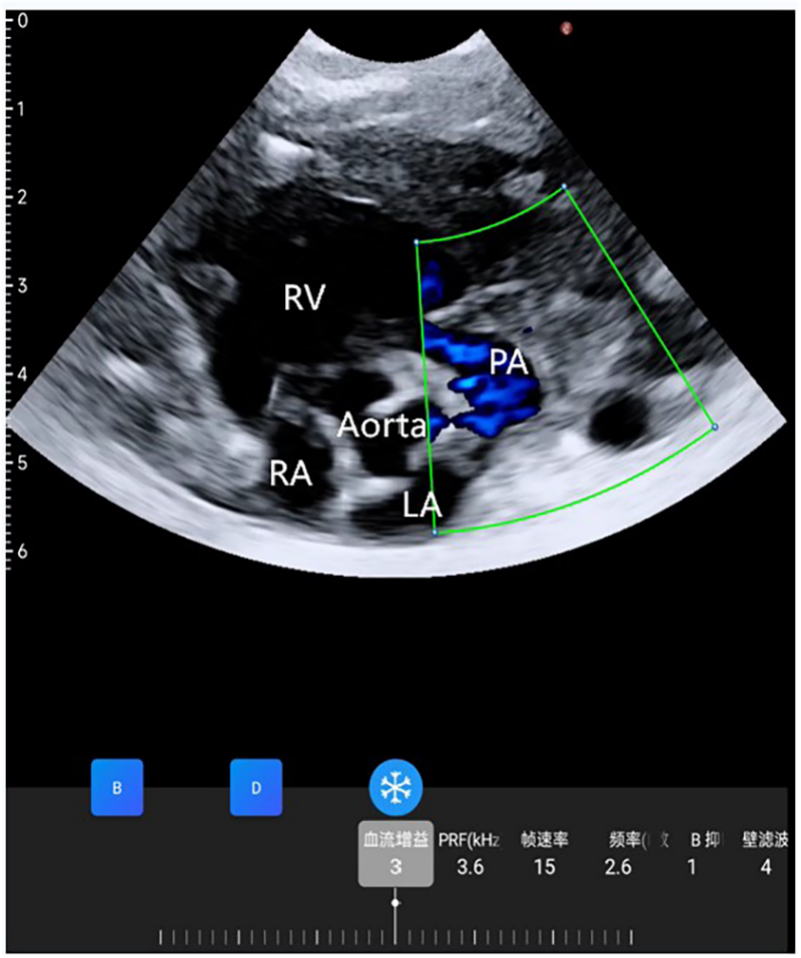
Wearable ultrasonic colour flow doppler examination between the aorta and pulmonary artery did not find a PDA.
